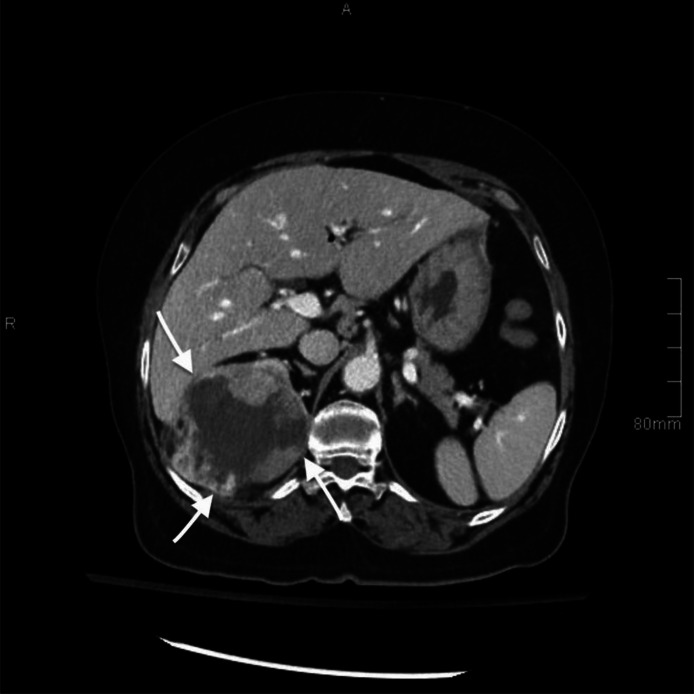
Axial contrast-enhanced CT image. The image shows a large mixed exophytic complex renal lesion in the right upper renal pole with an enhancing nodular component. The renal mass abuts the right hepatic lobe, diaphragmatic crus, and intercostal muscles (white arrows).
